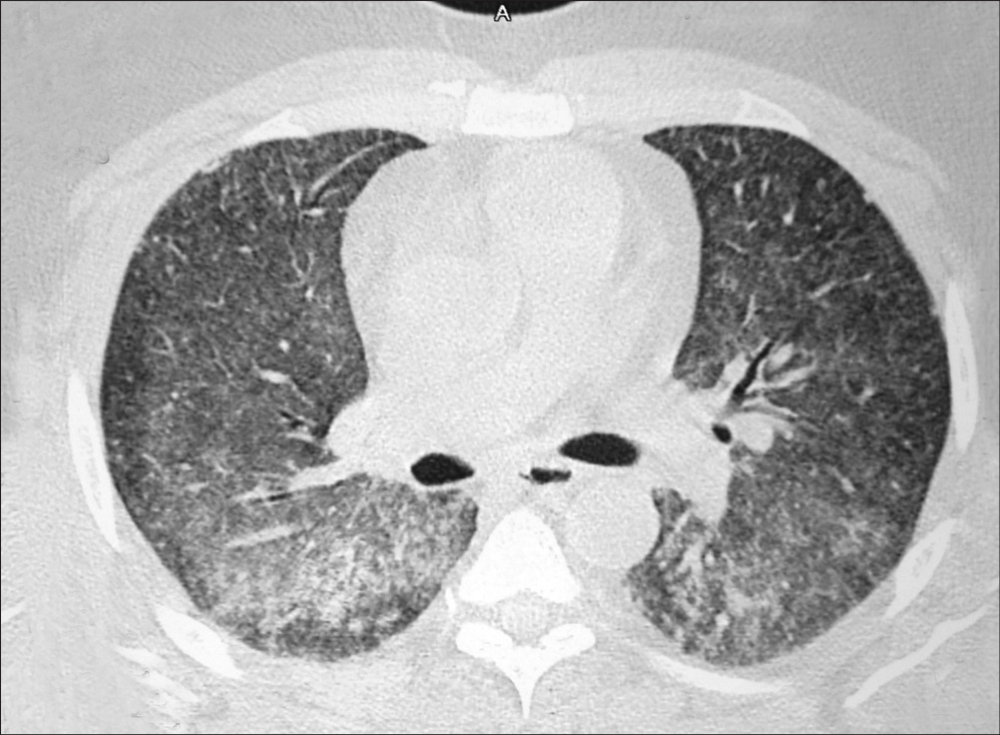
CT thorax showing diffuse ground glass opacities
